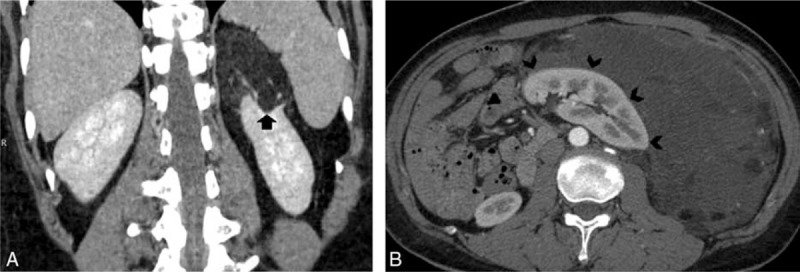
A, A 41-year-old man with exophytic renal angiomyolipoma and B, a 41-year-old female with retroperitoneal liposarcoma. Coronal contrast-enhanced multidetector computed tomography (CT), A, shows a fat-containing mass arising from the left kidney with a sharp parenchymal defect at the site of tumor contact (black arrow). Contrast-enhanced axial CT image, B, reveals a large fatty mass in the left perinephric space that partially engulfs the left kidney with mass effect displacing the abdominal contents to the midline and contralateral side. The interface between liposarcoma with kidney is smooth (black arrowheads).
